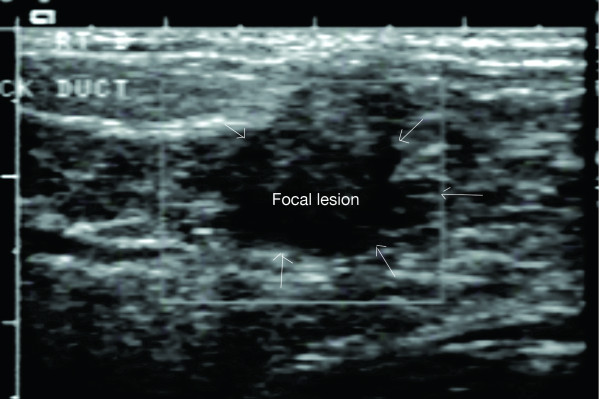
This palpable blocked duct appears as a small focal heterogeneous area with irregular margins on ultrasound.
